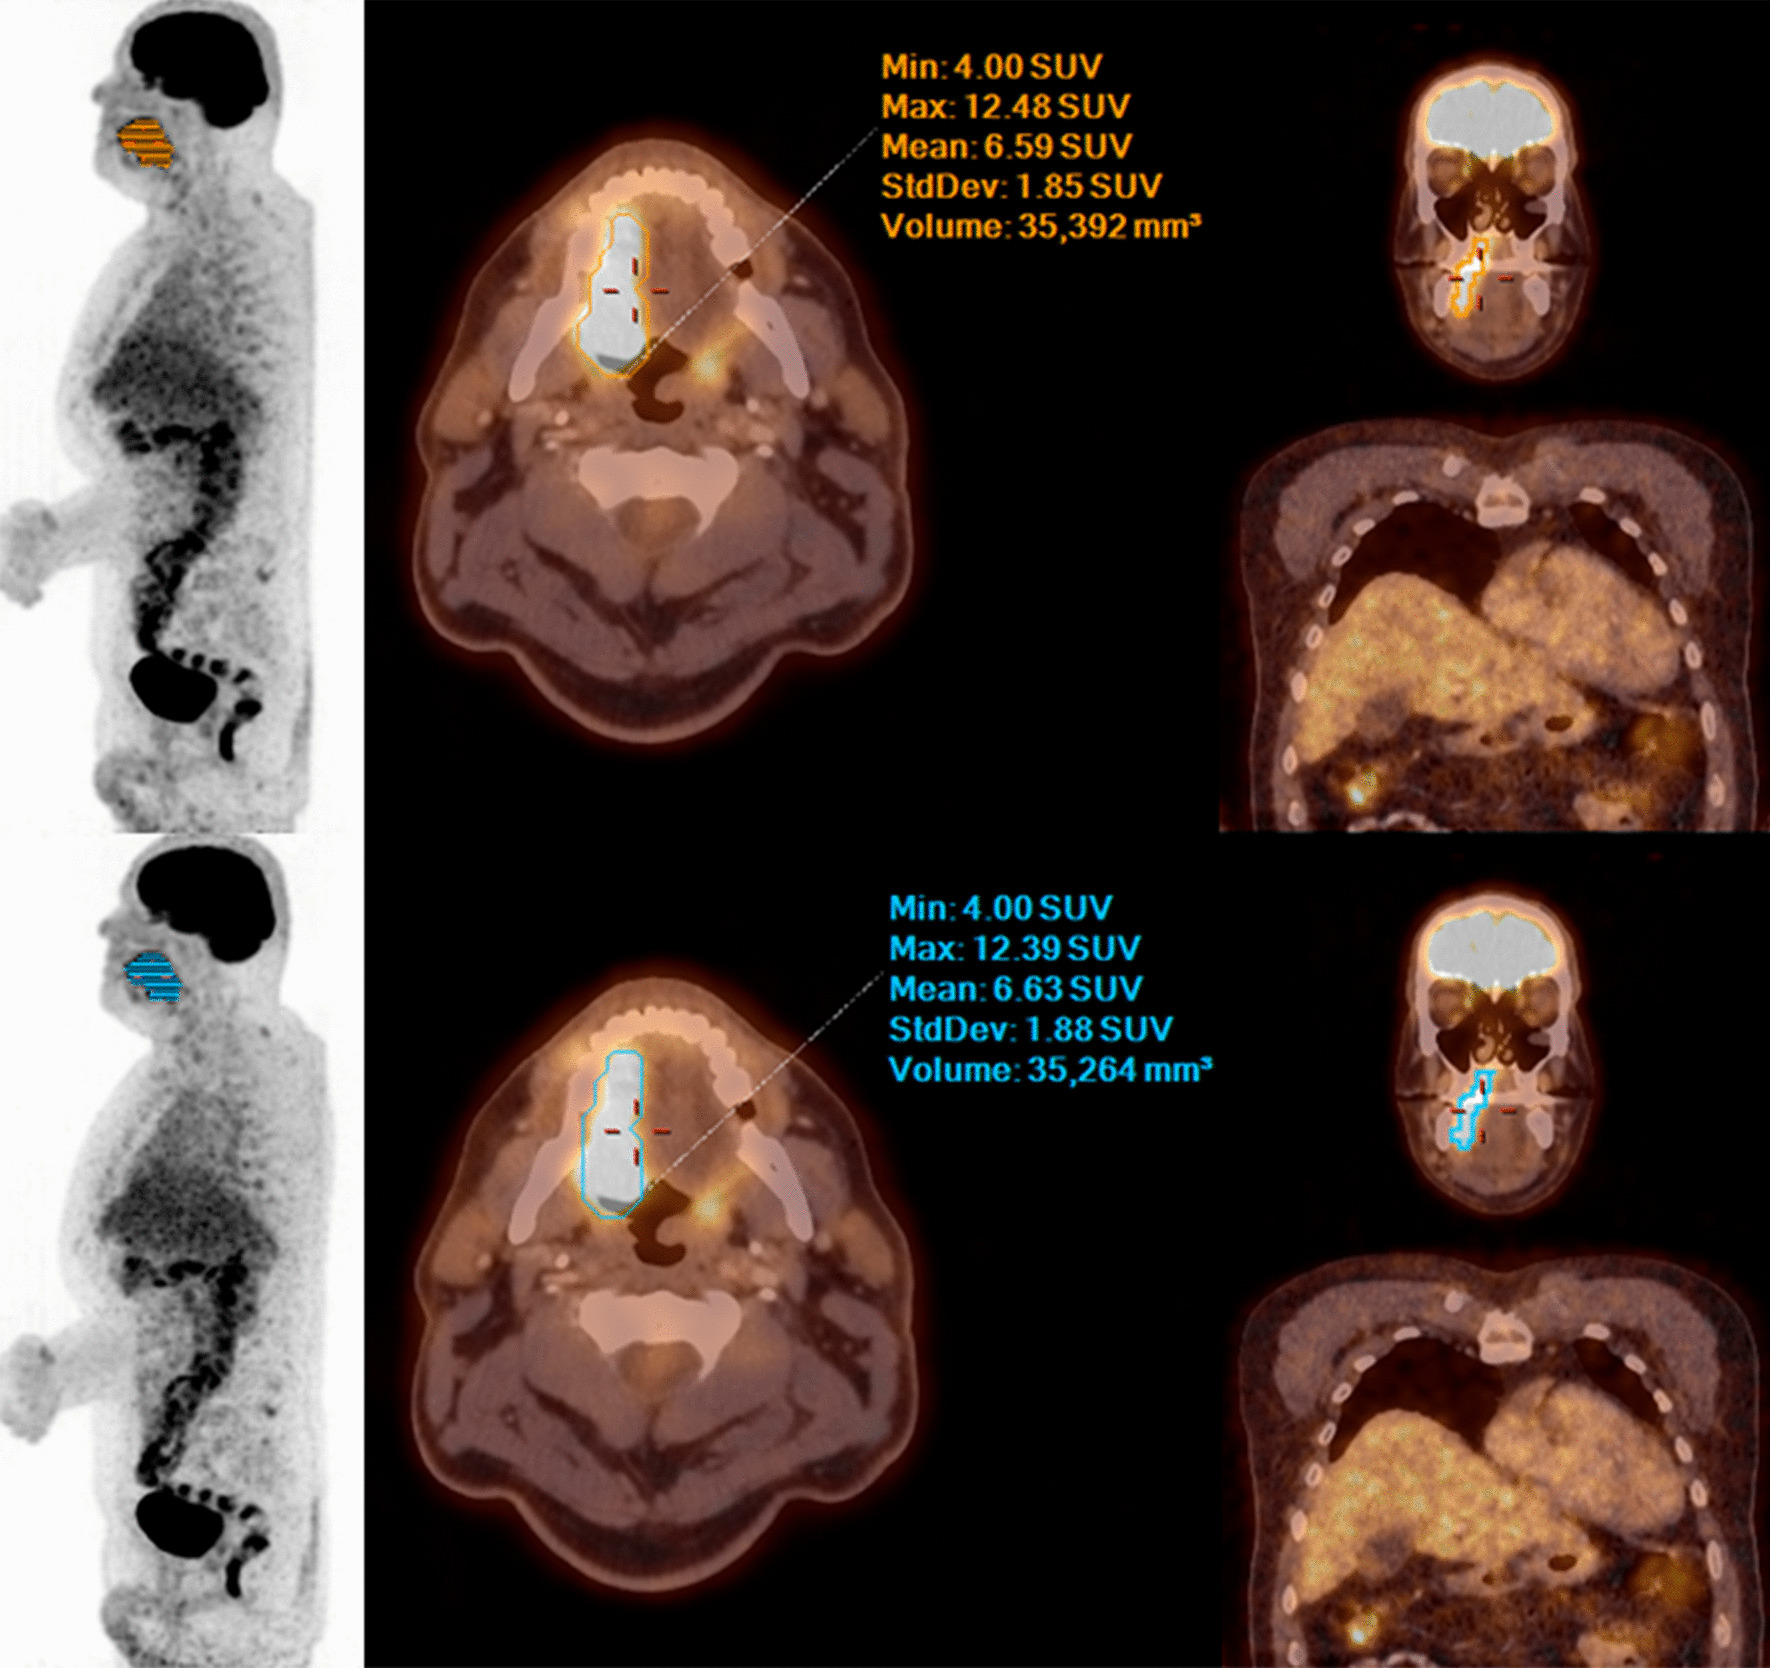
MIP, fused axial and coronal PET/CT images (from left to right) with 75 s (upper row )and 57 s (lower row) PET acquisition time per bed position of a 57-year-old male patient with 105 kg bw suffering from head & neck cancer—no significant difference in image quality, lesion detectability and quantification
